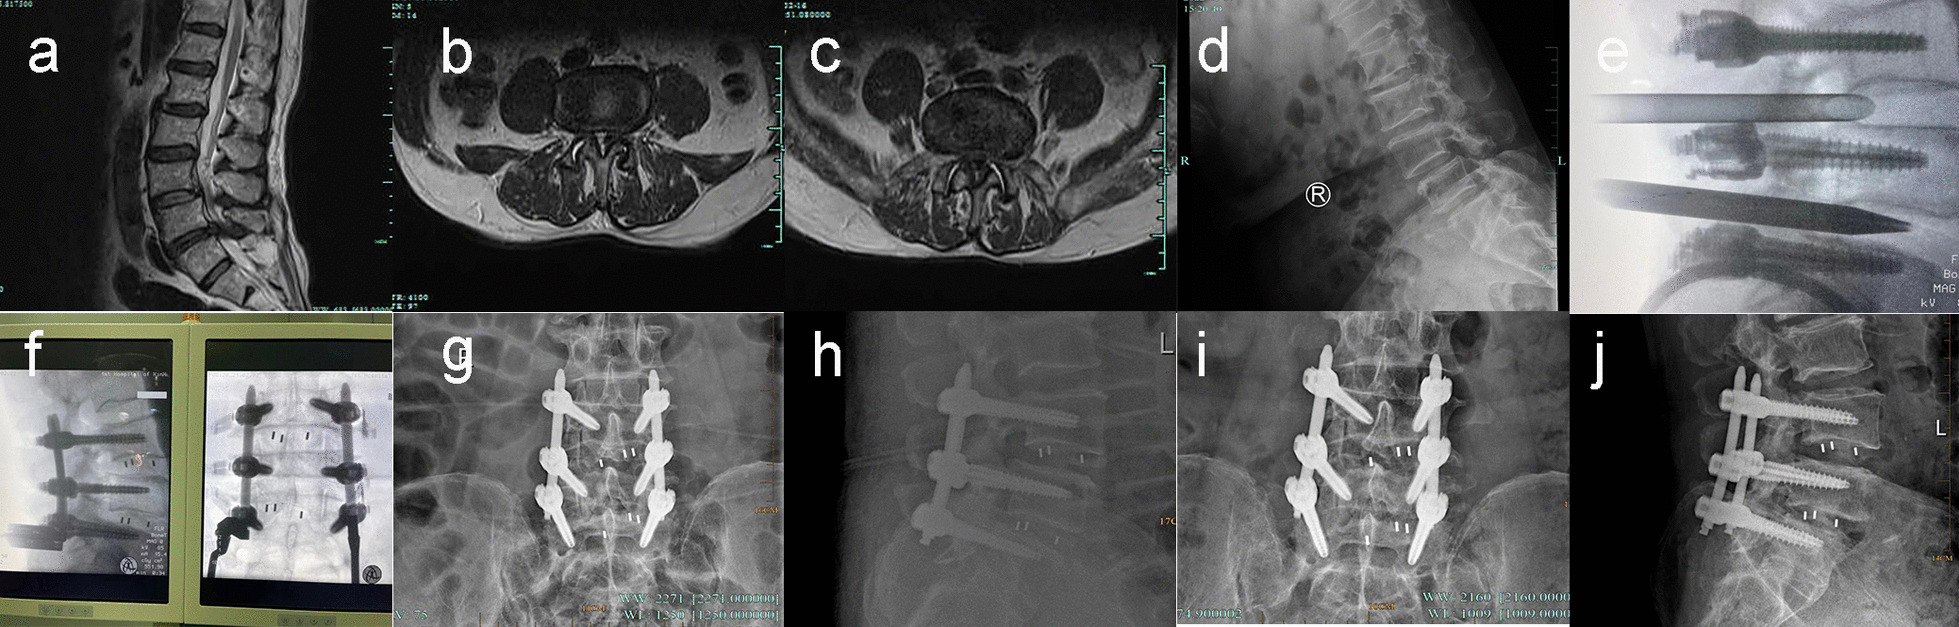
Typical case 5. An 82-year-old male with left hip pain and left lower limb numbness for over a year. Sagittal MRI shows disc herniation at L5/S1 level and spondylolisthesis at L4/5 level (a). Axial MRI show severe spinal stenosis at L4/5 and L5/S1 levels (b, c). Hyperextension X-ray demonstrates instability at L4/5 and L5/S1 levels (d). Finish the decompression and implant cage to intervertebral space (e, f). Anteroposterior and lateral X-ray one month after surgery (g, h). Anteroposterior and lateral X-ray 1 year after surgery (i, j)
